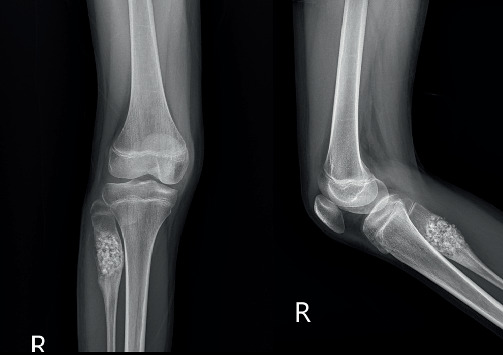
Plain radiographs at 2 months postoperation show successful incorporation of the allograft.
